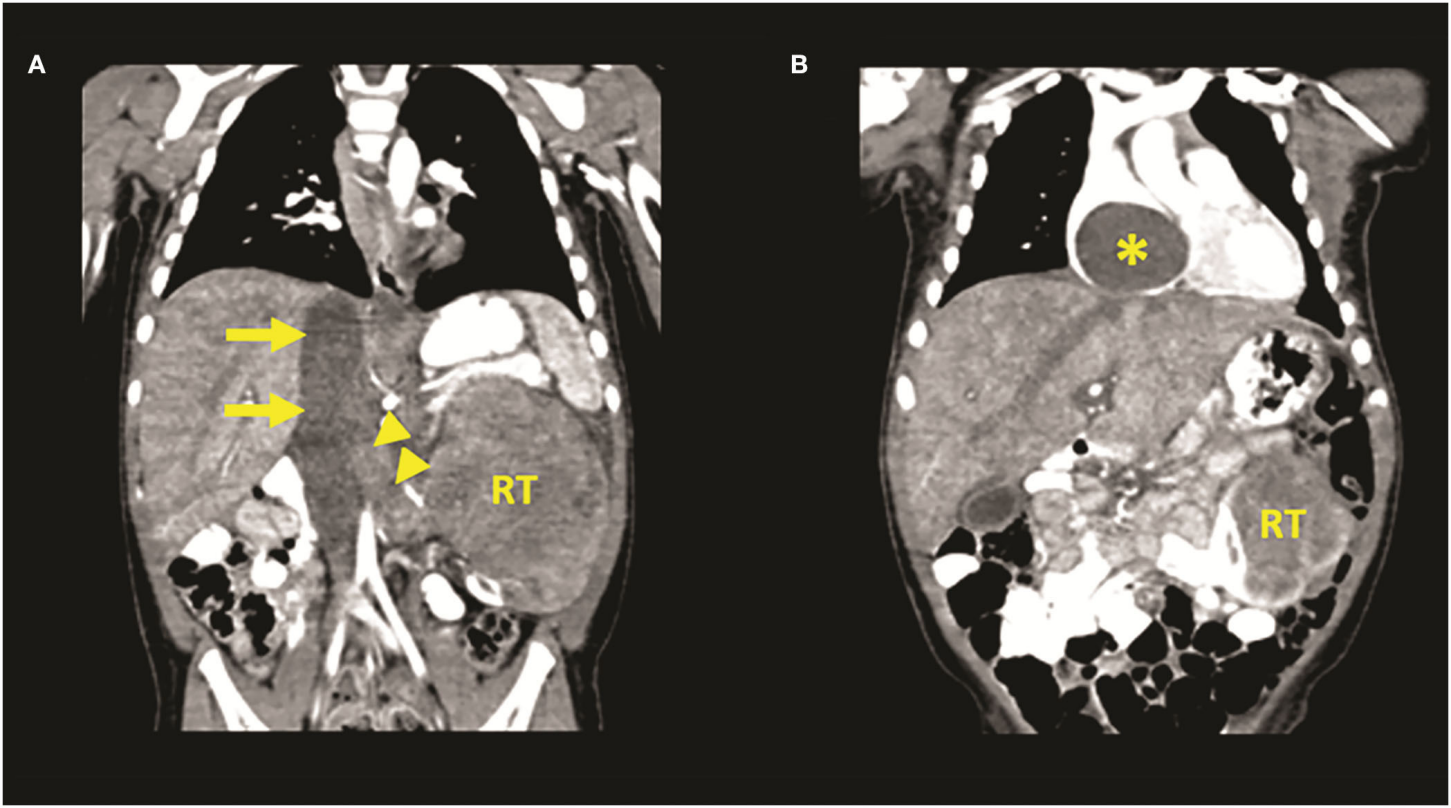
Venous phase of computed tomography (CT) angiography images (A,B) showing a left renal tumor (RT) with renal vein (arrowheads) and inferior cava vein tumor thrombus (arrows) extending into the right atrium (asterisk).
